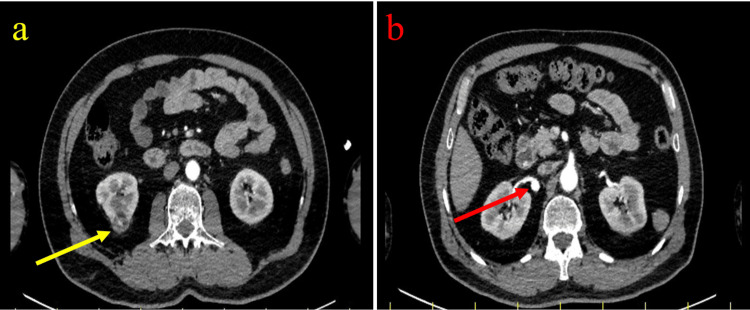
Axial contrast-enhanced CT images obtained in the arterial phase. (a) A 25-mm exophytic solid mass arising from the lower pole of the right kidney (yellow arrow). (b) A 1-cm saccular aneurysm located at the first bifurcation of the right renal artery (red arrow). Arterial-phase imaging allows clear delineation of both the renal mass and vascular anatomy, facilitating preoperative assessment and surgical planning.
